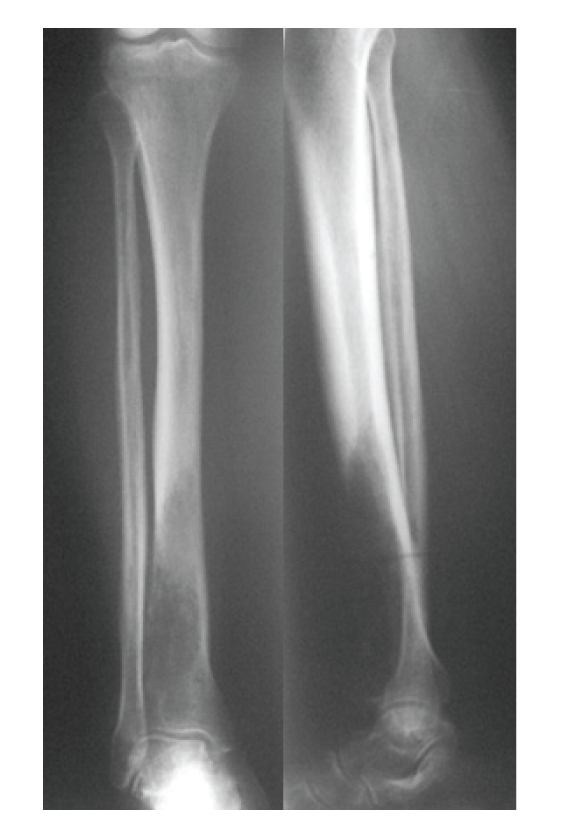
Radiograph showing an osteolytic lesion to the distal third of the tibia with cortical destruction.
